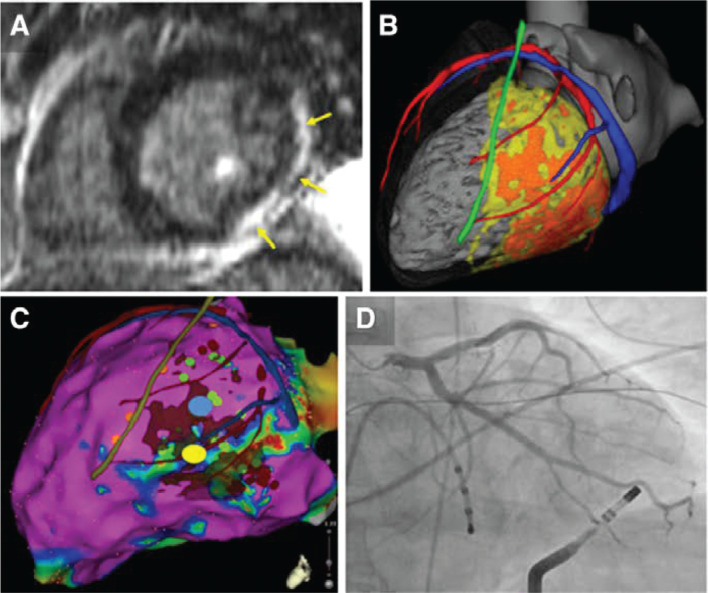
Example of image integration to assist ventricular tachycardia ablation in a case of myocarditis. A: Lateral and inferior left ventricular scar on cardiac magnetic resonance (CMR) (arrows). B: Patient-specific 3-dimensional model built from merged computed tomography (CT) (anatomy) and magnetic resonance imaging (scar) data. Cardiac chambers (gray), coronary arteries, and veins (in red and blue, respectively), left phrenic nerve (green), as segmented from CT, and dense scar and gray zone (in orange and yellow, respectively) as segmented from CMR. C: Epicardial bipolar voltage map with merged imaging model. Mid-diastolic potentials (not shown) are recorded during VT on an epicardial lateral left ventricular site (yellow dot in C). This potential target for epicardial ablation is far enough from the left phrenic nerve path derived from imaging (green line in C), which accurately matches sites of phrenic capture (orange dots in C). However, CT demonstrates the proximity of this site to a marginal branch of the circumflex artery on the registered imaging model. Confirmatory coronary angiography (D) demonstrates contact between the tip of the ablation catheter and the coronary artery. Reprinted with permission from Mahida S, Sacher F, Dubois R, et al. Cardiac imaging in patients with ventricular tachycardia. *Circulation*. 2017;136(25):2491–2507.
